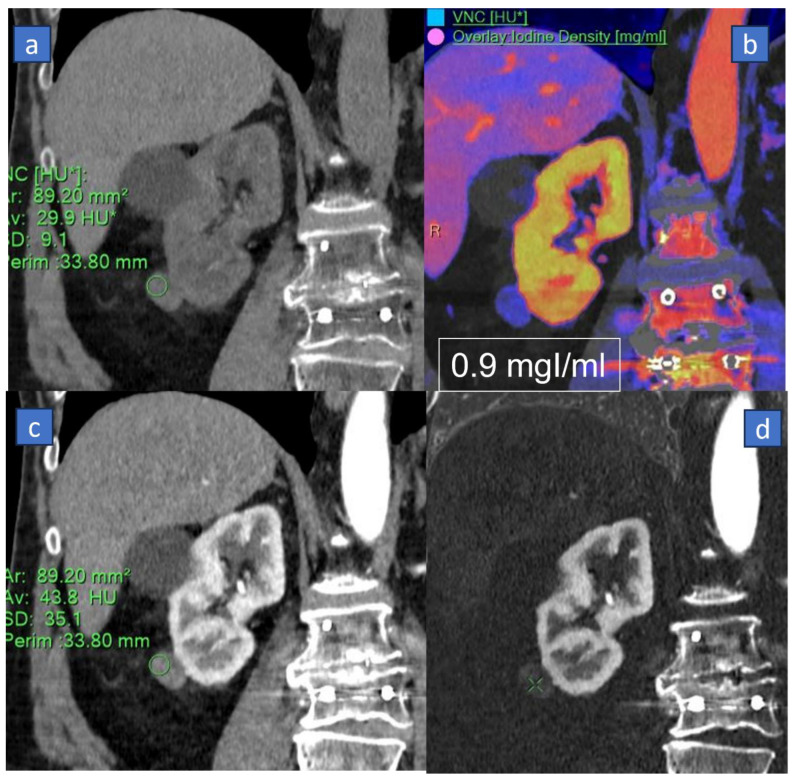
Sixty-eight-year-old woman suffering from incidental surgically proven papillary carcinoma of the right kidney. On the coronal reconstructed conventional baseline CT, (a) a small exophytic inferior polar nodule of the right kidney can be recognized (circular region of interest) with solid density (29.9 HU). On the corresponding iodine density superimposed on the virtual non-contrast image on the coronal plane, (b) the lesion shows a clear iodine uptake (0.9 mgI/mL), with different behavior compared to the simplex cyst located on the mid third of the same kidney. On the corresponding coronal contrast enhanced CT image, (c) the lesion demonstrates equivocal enhancement (43.8 HU), and the density could be affected by artifacts due to metal spine fixation. The qualitative assessment of the corresponding coronal subtracted image (d) shows only a mild equivocal enhancement.
